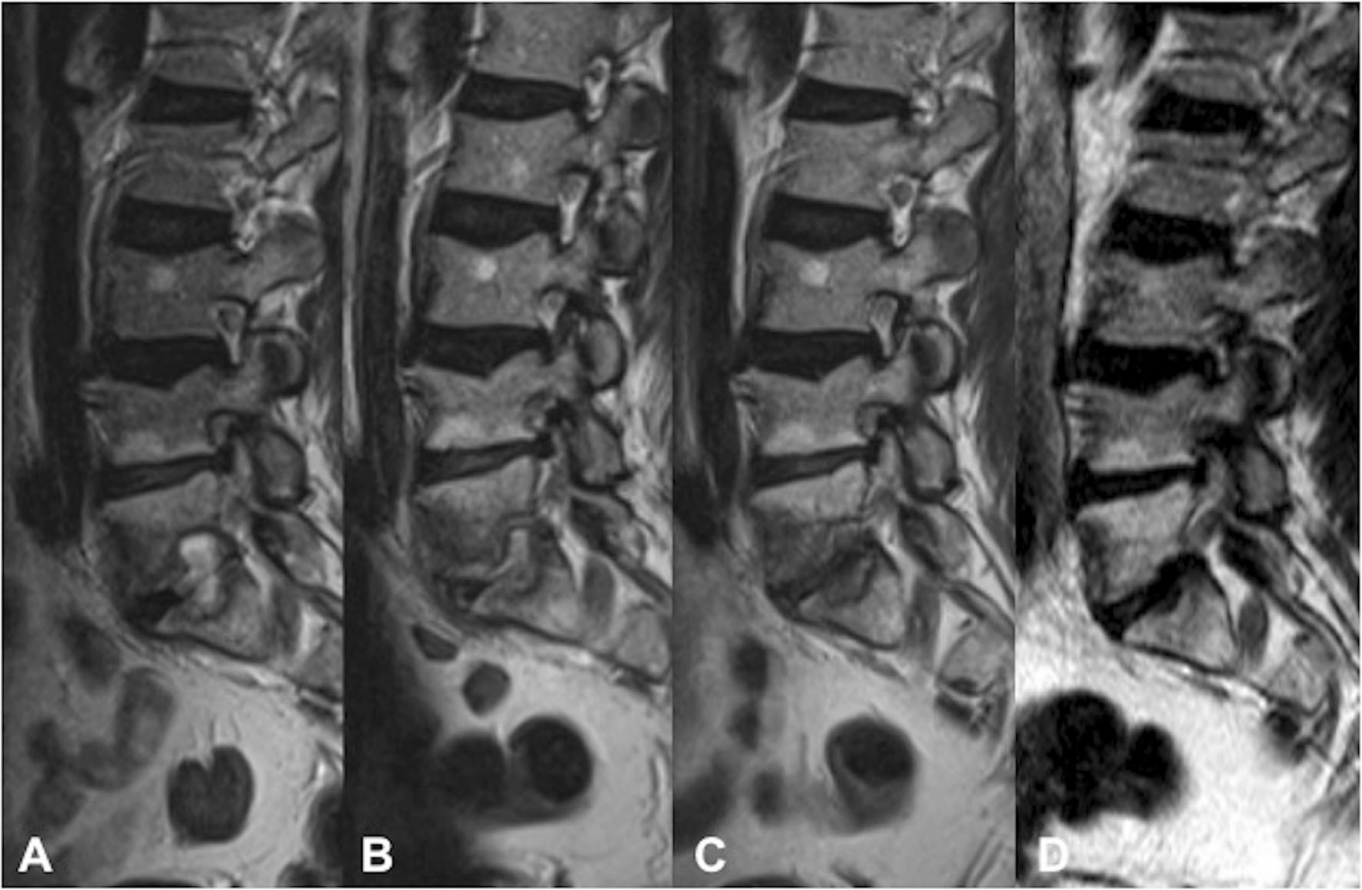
A 59-year-old man (patient 4). Sagittal T2 images from serial MRI studies demonstrating gradual resolution of an L5/S1 disc abscess extending into the adjacent endplates. a 3 months following EVAR, b 6-week interval following the initial MRI, c 3-month interval and d 14-month interval
